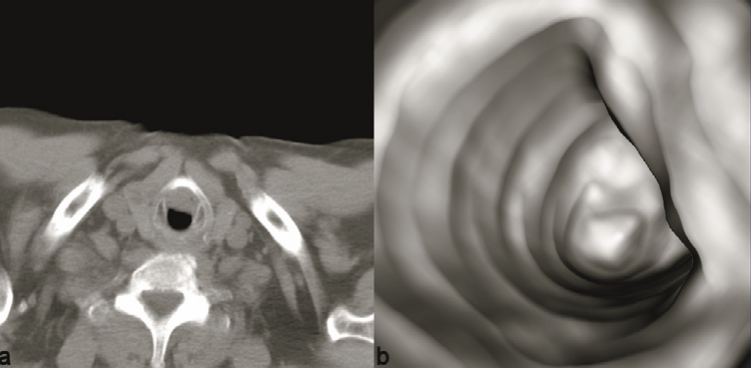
Tracheal stricture after invasion of adjacent thyroid cancer. Axial CT shows parenchymal irregularity and enlargement of the right thyroid lobe. The mass has eroded the right lateral tracheal wall and extended intraluminal to the trachea. Reformatted virtual endoscopy images portray the eccentric tracheal stenosis with an excellent 3-dimensional perceptive.
